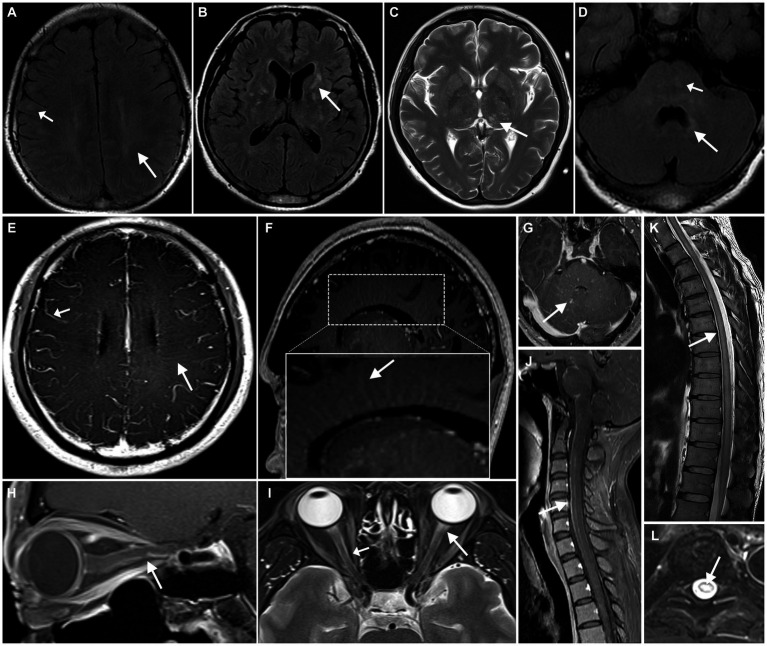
Neuroimaging findings with autoimmune GFAP astrocytopathy. cerebral parenchymal lesions can be observed in the subcortical white matter (A, short arrow), periventricular regions (A, arrow), basal ganglia (B), thalamus (C), brainstem (D, short arrow), and periventricular regions of the fourth ventricle (D, arrow). MRI enhancement shows enhancement of the leptomeninges (E, short arrow) and radial linear enhancement surrounding the lateral ventricles (E,F, arrow) and fourth ventricle (G). A hyperintense lesion is observed in the anterior portion of the optic nerve on T2WI (I, arrow), with the optic nerve sheath showing hyperintensity on T2WI (I, short arrow) and enhancement on contrast-enhanced MRI (H). Spinal cord shows longitudinally extensive transverse myelitis (K,L), with linear enhancement seen in the meninges and central canal of the spinal cord (J).
